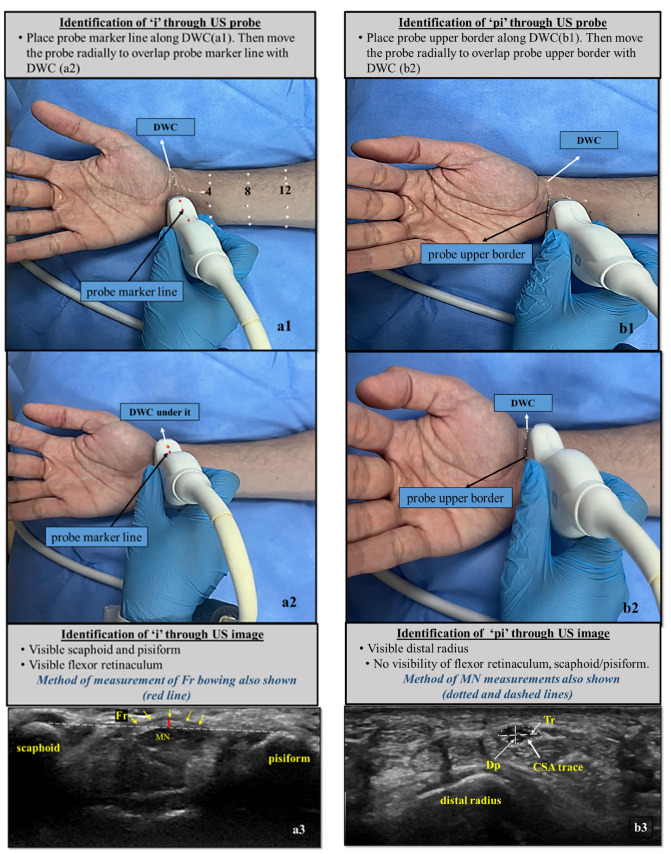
Pictures of wrist with reference markings of the sites where ultrasound examination of median nerve was performed, i.e., at ‘i’, ‘pi’, 4,8,12 cm from DWC (a1–b2). Corresponding sonographic appearance of MN at ‘i’ and ‘pi’, method of MN measurements taken and identification of location are also shown (a3,b3). Multiple downward arrows = flexor retinaculum, dashed horizontal line in (a3) = reference line drawn between scaphoid and pisiform to measure bowing (vertical red line) of Fr, dashed horizontal line in (b3) = Tr dimension of MN, dashed vertical line in (b3) = Dp of MN, dotted circle in (b3) = CSA of MN. *US = ultrasound, DWC = distal wrist crease, Fr = flexor retinaculum, CSA = cross-sectional area, Tr = transverse, Dp = depth, carpal tunnel inlet = ’i’, proximal to carpal tunnel inlet ‘pi’, MN = median nerve.*
